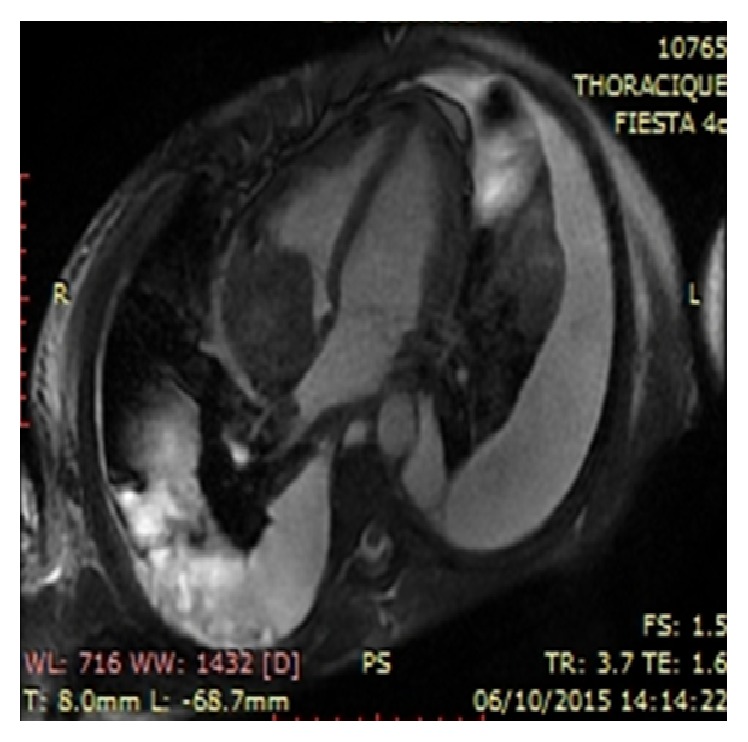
Cardiac MRI, thrombosis occupying most of the right atrium. Cross section of the cardiac MRI through the atria, the white ring shows the massive thrombosis at the right atrium.
